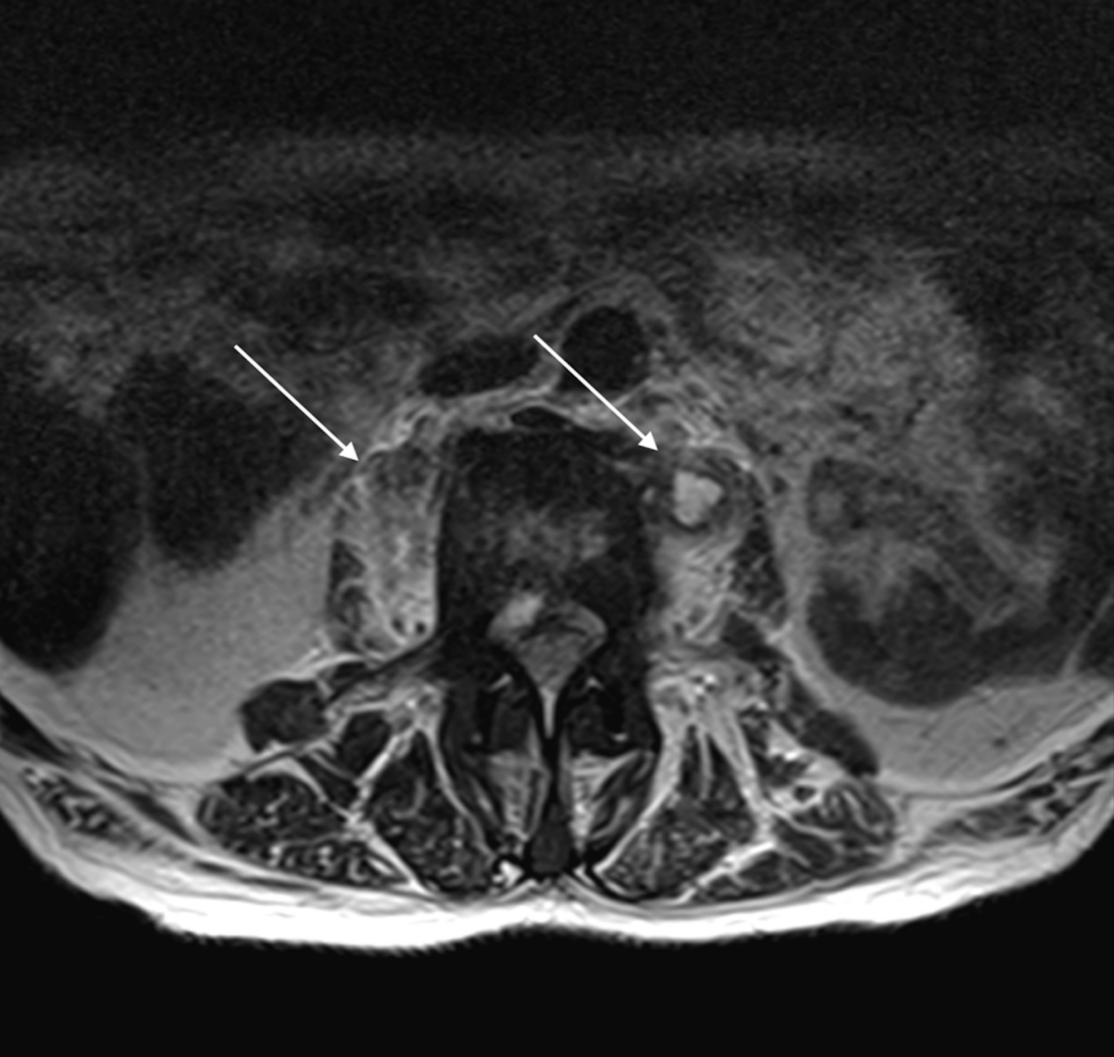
Magnetic Resonance Imaging with Contrast of the Lumbar Spine Axial image of the lumbar spine revealing multiple abscesses (see arrows) in the psoas muscles with prevertebral inflammation
